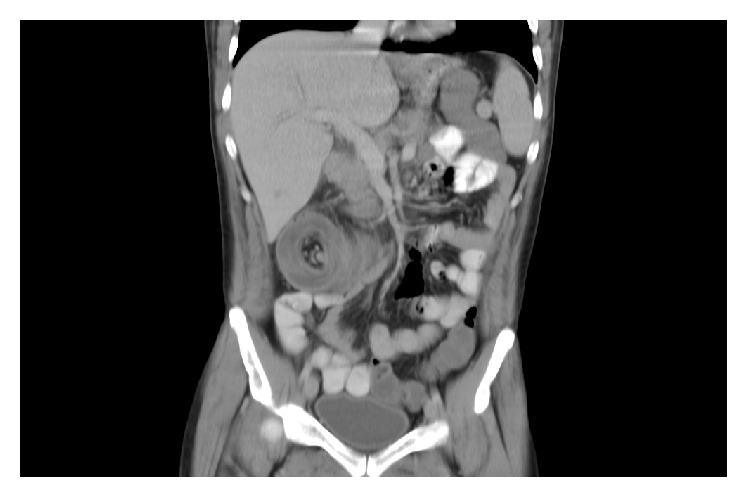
Coronal reconstruction of contrast enhanced abdomen and pelvis CT showing long segment of ileocolic intussusception with classic target/“doughnut” sign.
